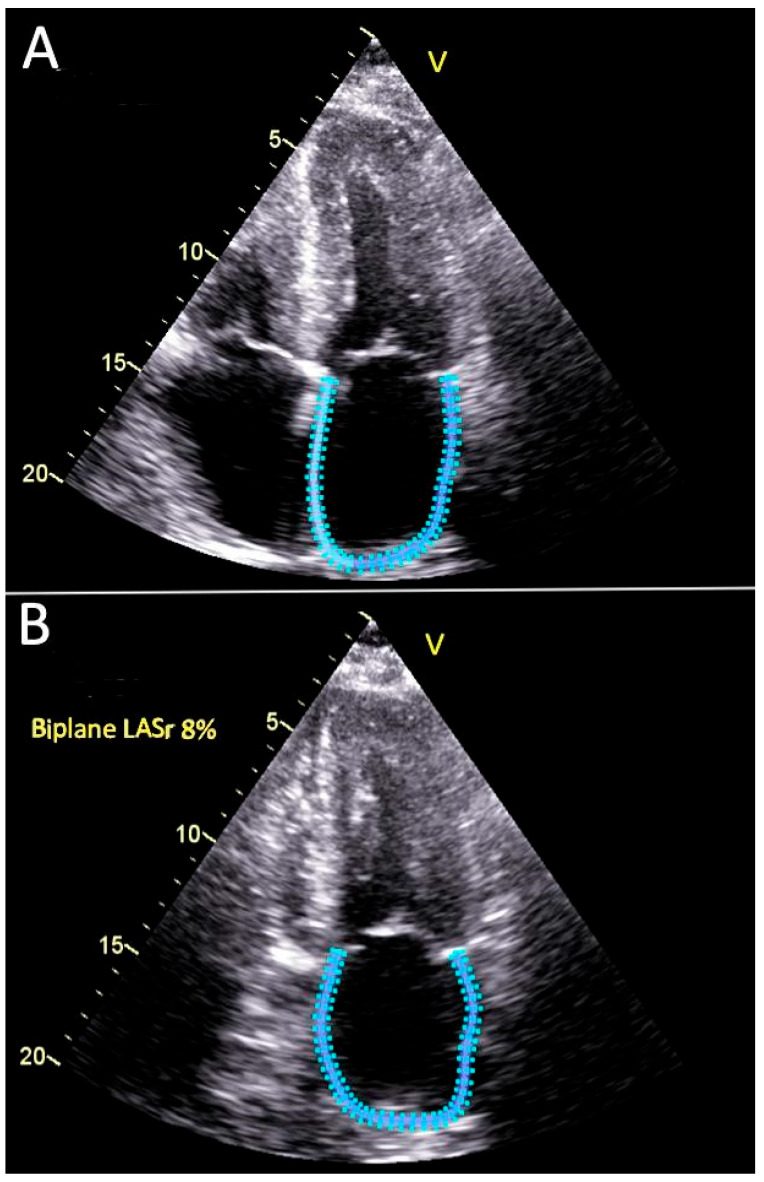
Automated measurement of biplane average peak left atrial reservoir strain. (A) Apical 4-chamber view. (B) Apical 2-chamber view. The left atrial endocardial border is tracked using an automated speckle tracking algorithm. The calculated biplane left atrial reservoir strain value is shown in panel B, LASr = 8%.
